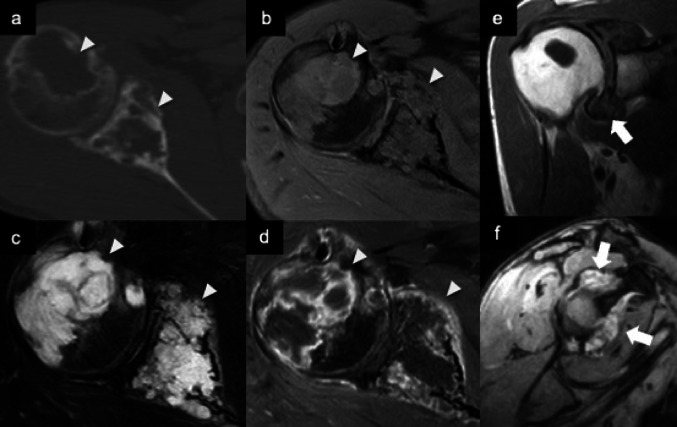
Synovial chondrosarcoma. Axial CT bone image (a) shows osteolytic lesion (arrowheads) of the humeral head and scapula. Axial fat-suppressed T1-weighted MR image (b) demonstrates lobulated intramedullary lesions in both scapula and humerus (arrowheads). Axial fat-suppressed T2-weighted MR image c reveals high signal intensity of the lesion, and fat-suppressed postcontrast T1-weighted MR image (d) shows peripheral gadolinium enhancement suggesting chondroid nature (arrowheads). Sagittal T1-weighted MR image (e) and oblique sagittal T2*-weighted MR image (f) obtained 6 years earlier revealed a diffuse synovial lesion of the intra-articular space (arrows)
